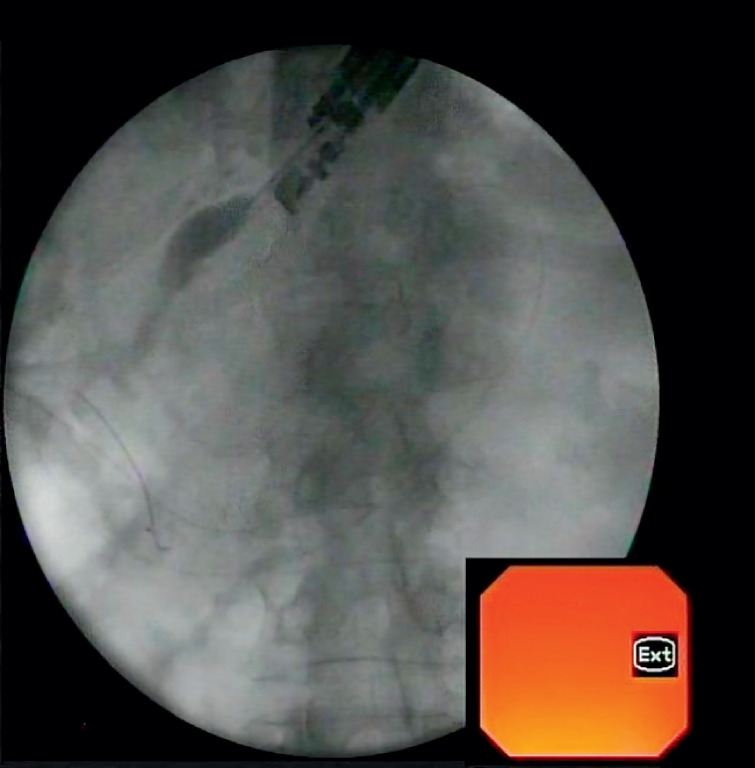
Endoscopic ultrasound-guided rendezvous technique.
